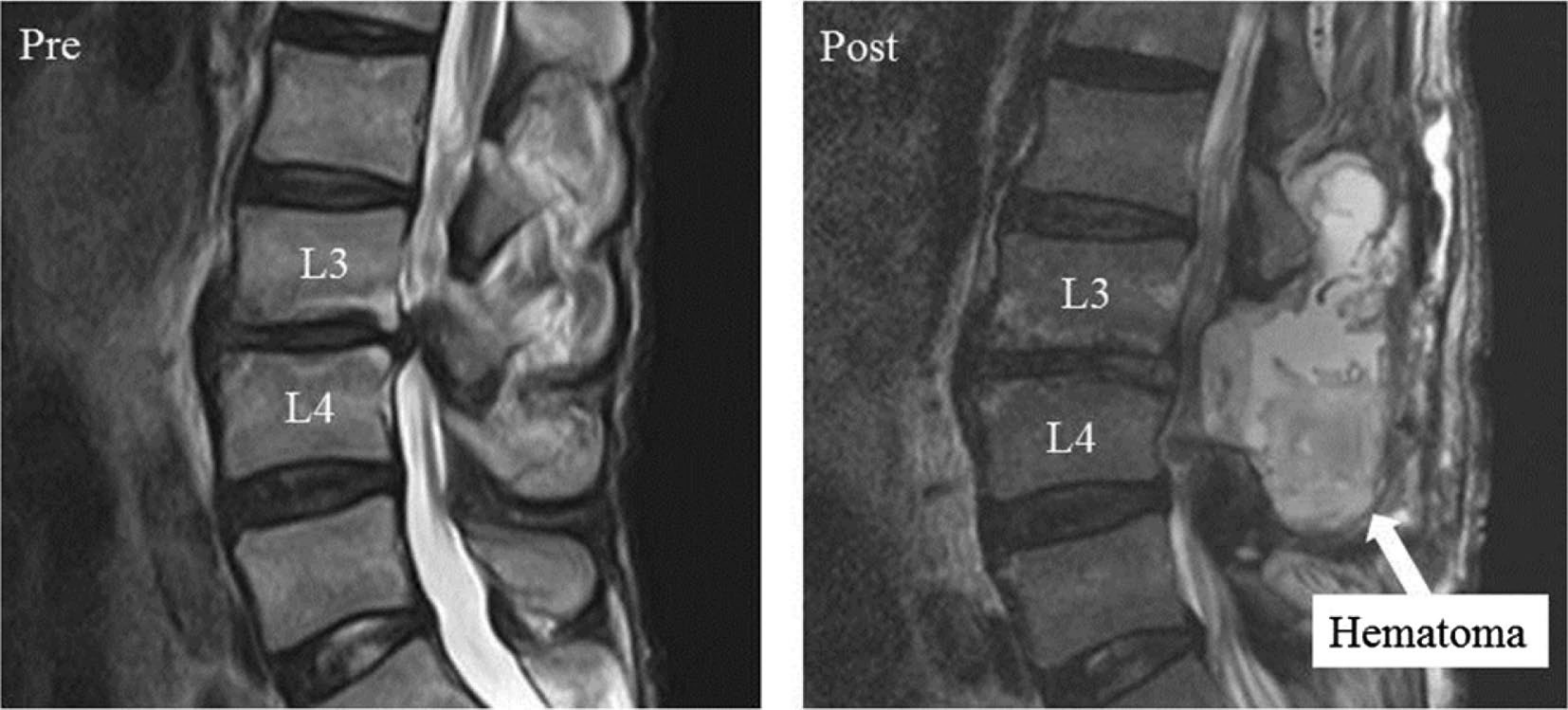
Magnetic resonance imaging of the lumbar spine pre- and post-surgery
